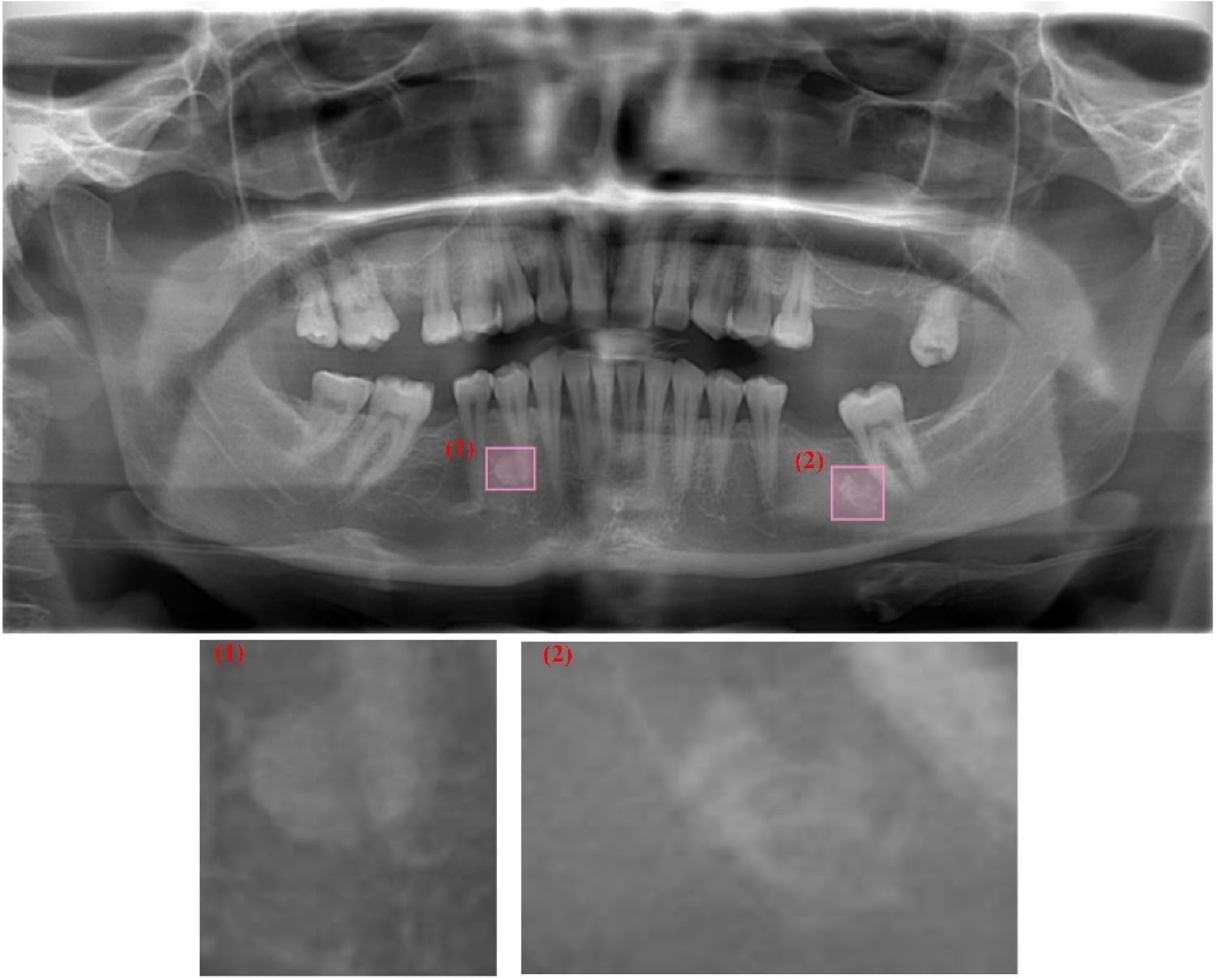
Two annotated IO on high-contrast panoramic radiography and their zooming for close monitoring of pattern changes.
